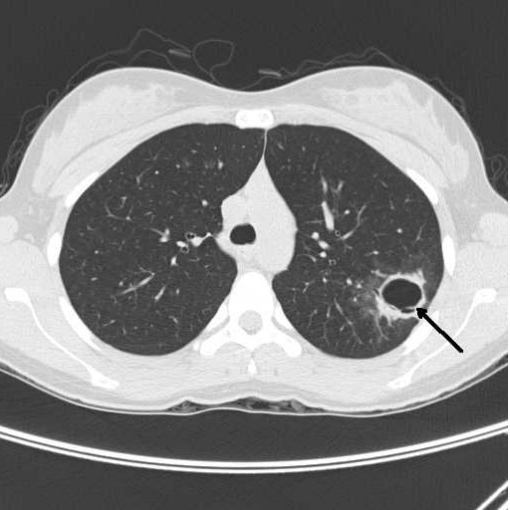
Chest CT showed a 3 cm cavitary lesion in the left upper lobe with surrounding consolidation.
